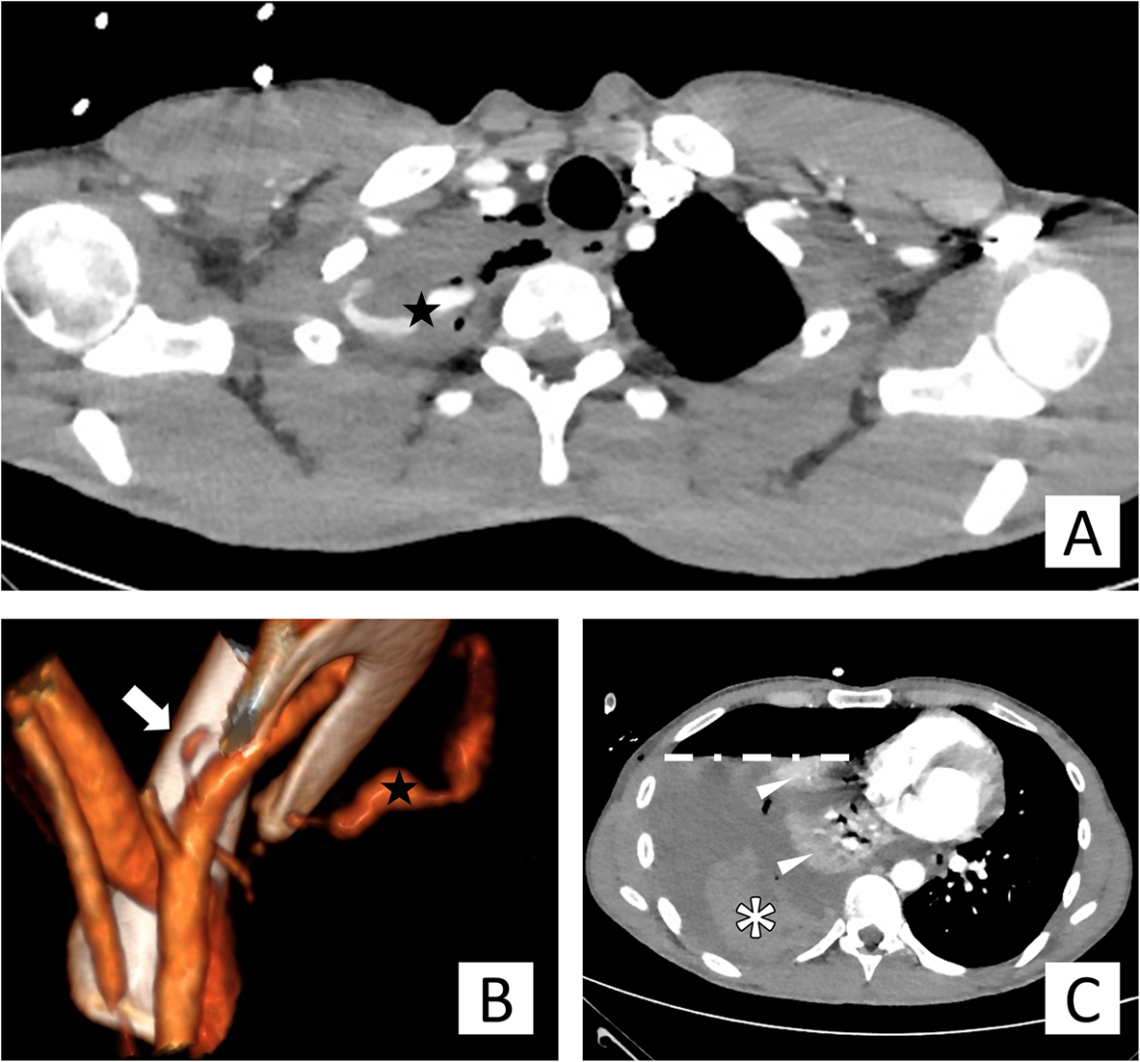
CT scan with contrast medium and Volume rendering of the active bleeding. The Axial CT scan (a) shows the blushing of contrast media in the right lung apex (star) that crosses into a blood clot. The volume rendering reconstruction (b) with a back view of the subclavian artery highlights its passage between the clavicle and the first rib. The three-dimensional reconstruction shows also, two vessels arising from the superior side of the artery, the thick white arrow points the thyrocervical trunk while the star indicates the outflow of contrast media. In the CT slice (c) are detectable: the right lung collapse (arrowheads), the presence of an air-fluid level (dashed line) with a dishomogeneous avascular irregular-shaped mass in the posterior pleural cavity as hallmark of a haemo-pneumothorax with huge coagulated blood (asterisk) and, lastly, the consequent mediastinal left-shifting
